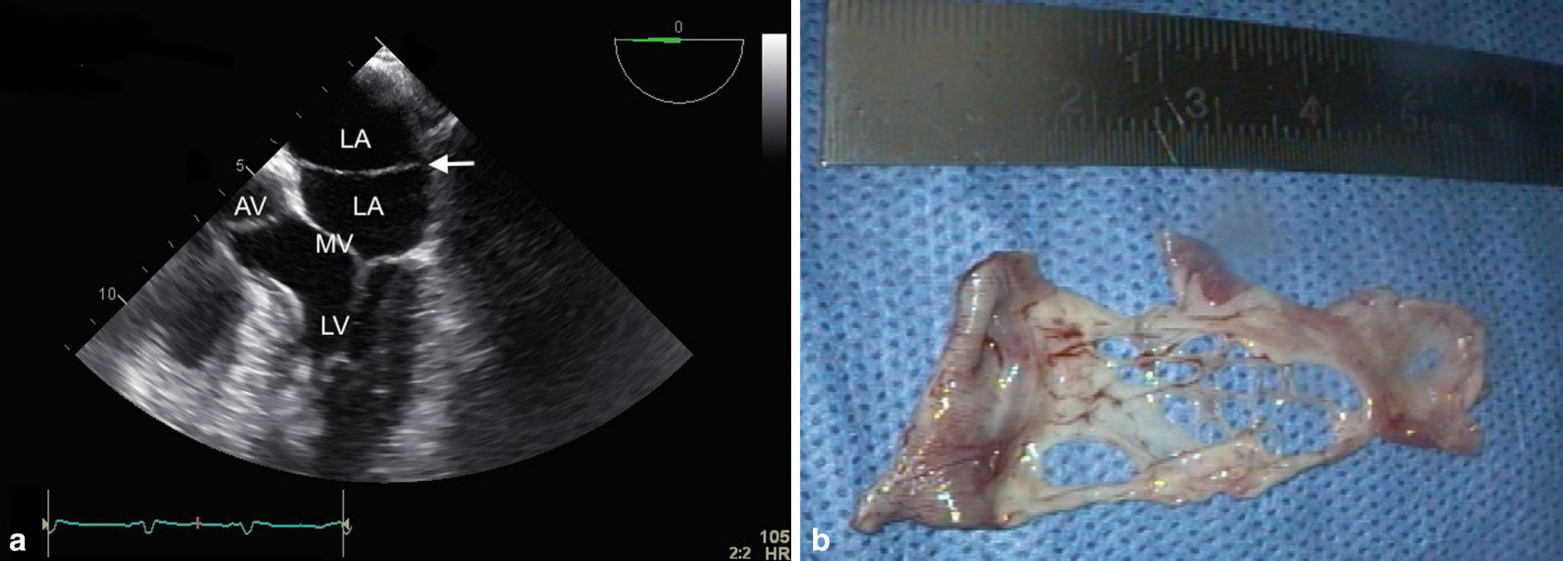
a Transoesophageal echocardiographic image of the left atrium (*LA*), the left ventricle (*LV*), aortic valve (*AV*) and the mitral valve (*MV*). The arrow points to the fibromuscular membrane. b Postoperative image of the resected fibromuscular membrane with multiple fenestrations
